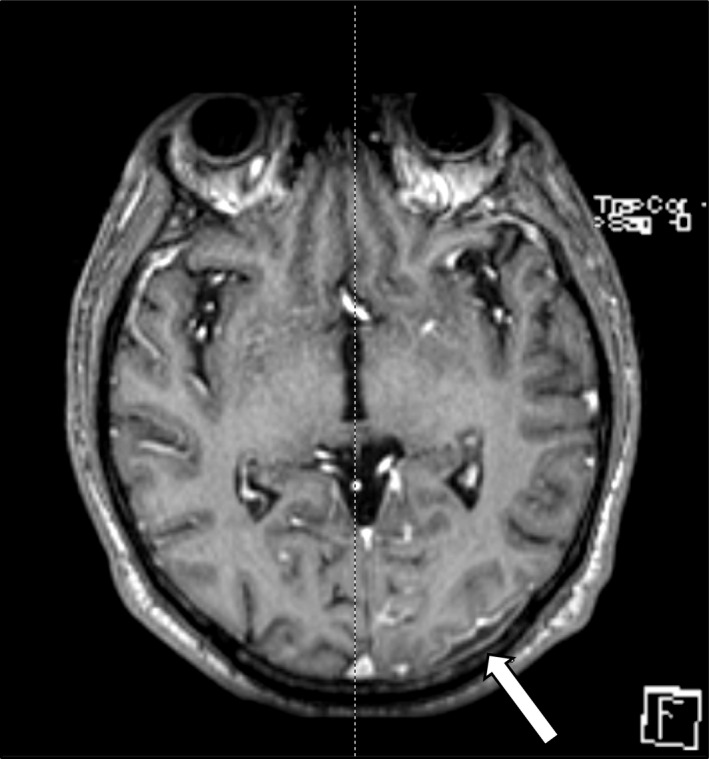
MRI brain with 11mm left occipital fluid collection consistent with a subdural empyema
